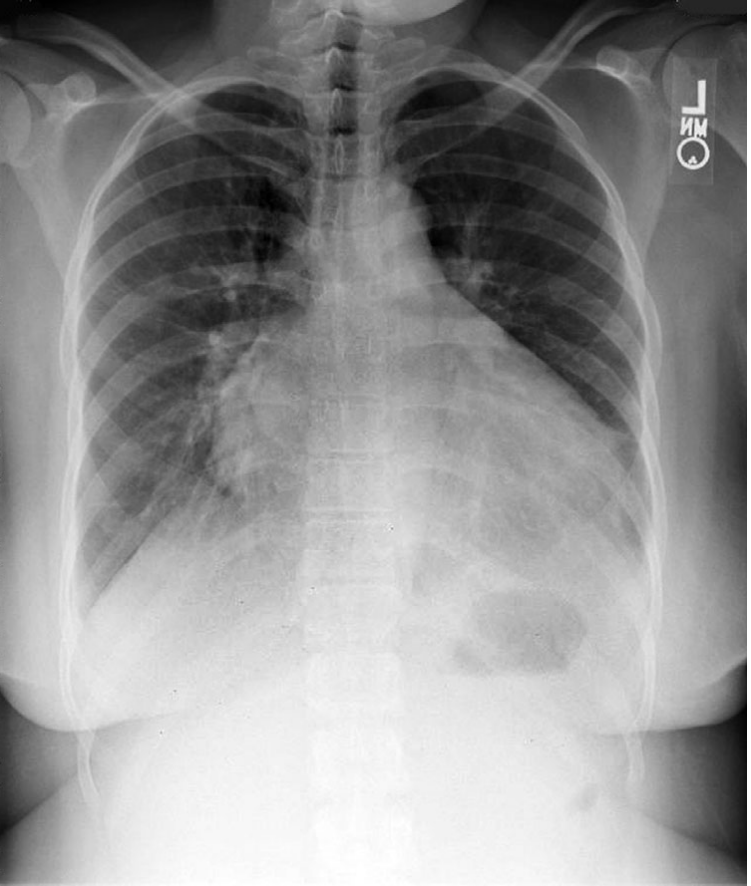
Chest x-ray showing moderate cardiomegaly and prominent bibasilar interstitial markings.
